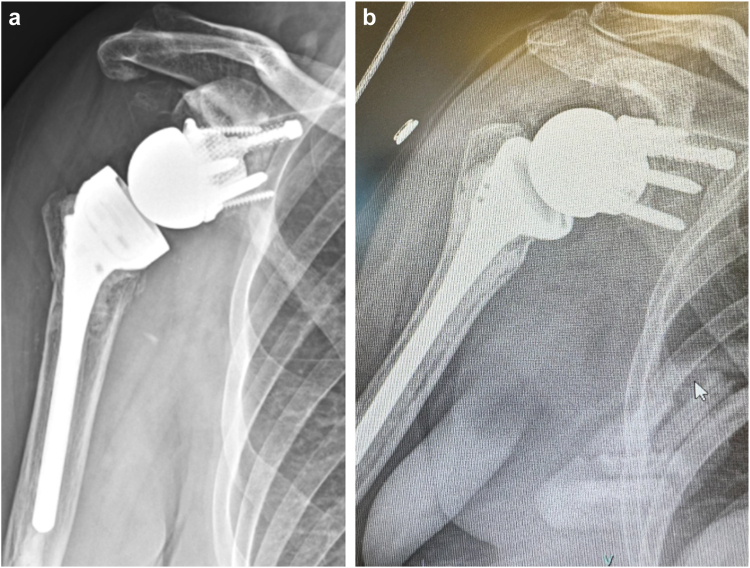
(a) Immediate post-operative radiograph demonstrating a combination of 4.0 mm and 5.0 mm screws. (b) Immediate post-operative radiograph demonstrating fixation with all 5.0 mm locking screws.
